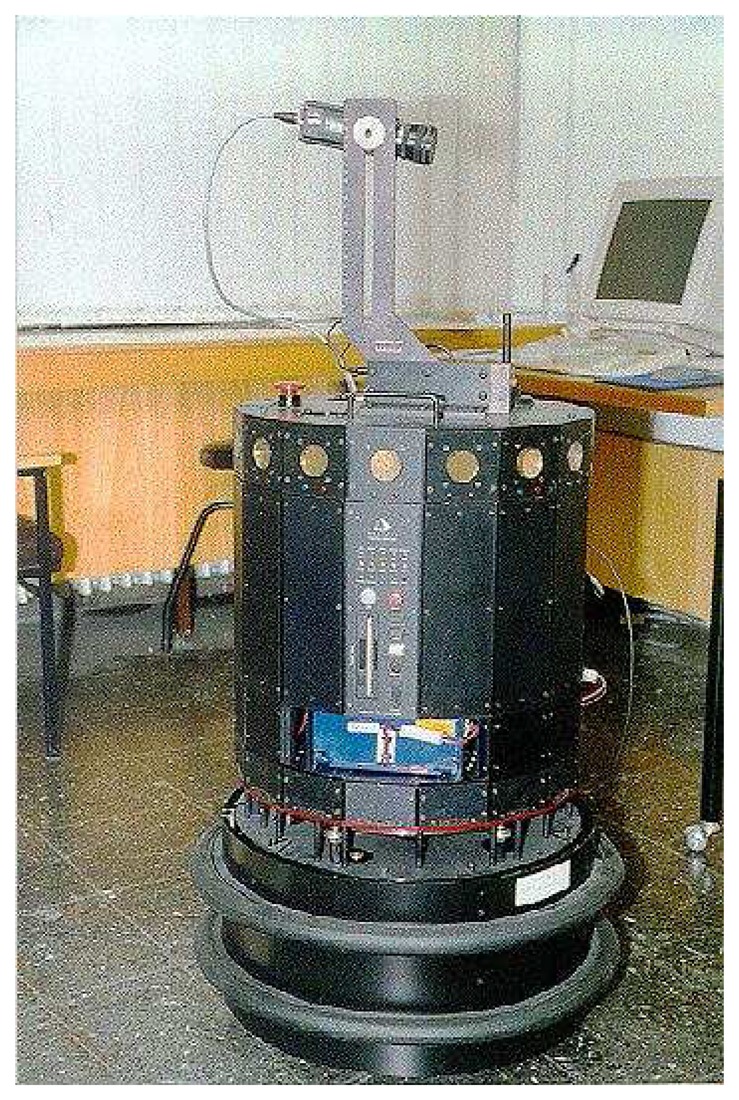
Nomad 200 mobile robot. The ring of ultrasonic sensors can be seen close to the top rim of the turret, and the structured-light laser system is seen pointing rightwards on top.
